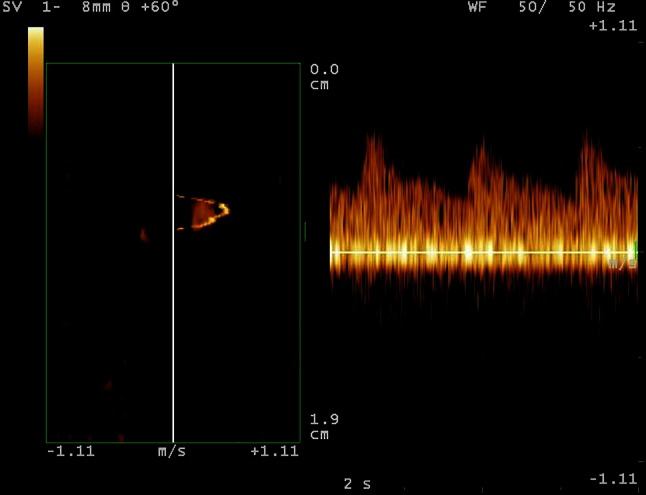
Blood flow profile in the acral finger arterioles obtained by the algorithms of multigate spectral Doppler in a healthy subject. The spectral flow profile in the acral finger arterioles is displayed in *left panel* and corresponding flow wave of pulsed Doppler is shown in *right panel*
